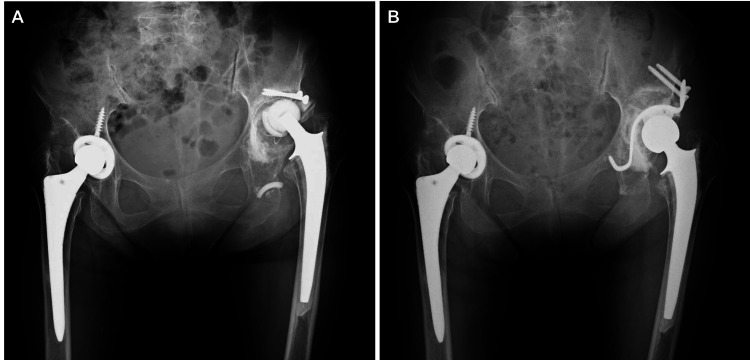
Radiographic images (A) Preoperative and (B) postoperative radiographic images with an anteroposterior view of the bilateral hip joint. For the loosening of the acetabular component with a bone defect, revision total hip arthroplasty was performed with acetabular reconstruction.
